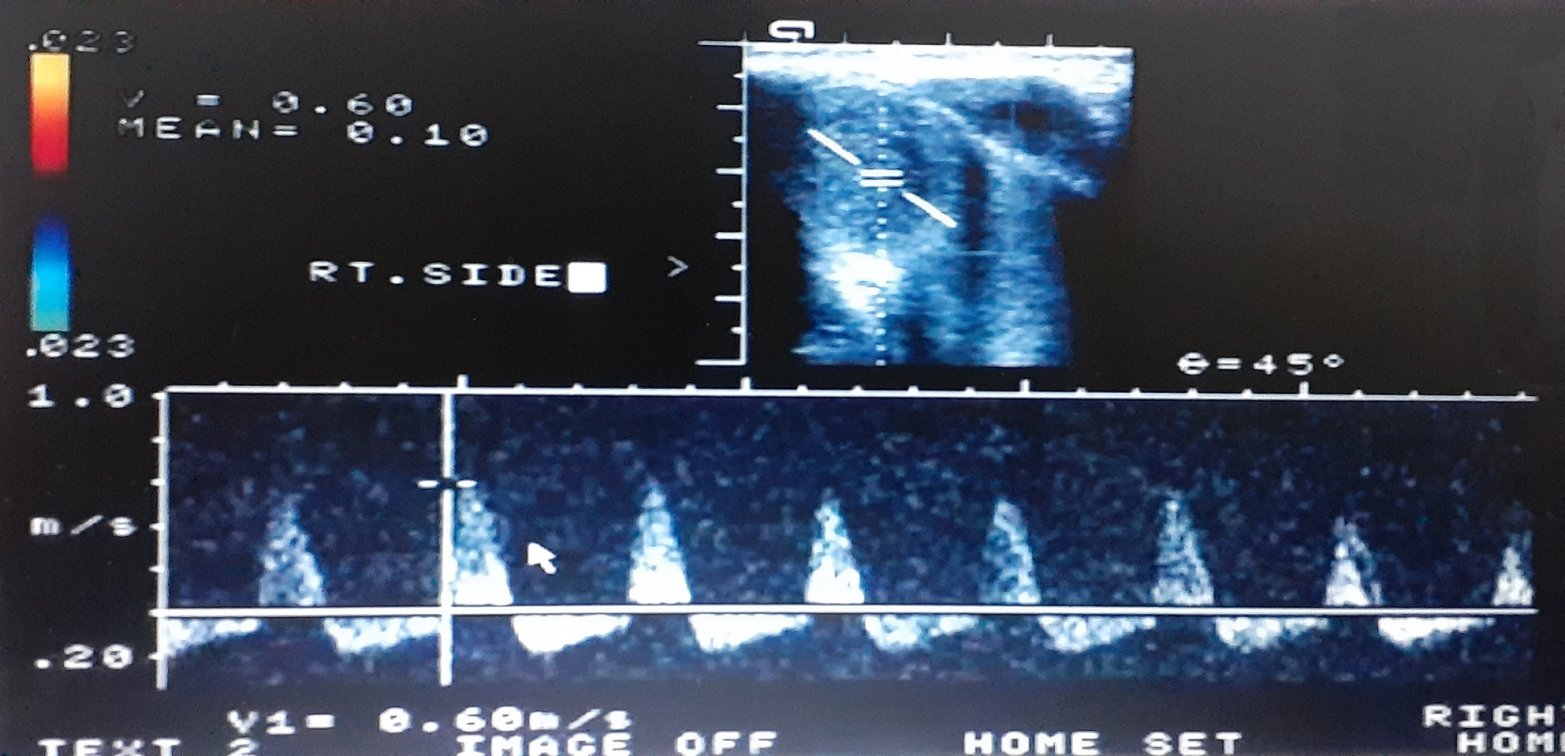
Penile Doppler ultrasound shows a normal arterial flow of the penis in an infant with high‐flow priapism, without fistula appearance.
